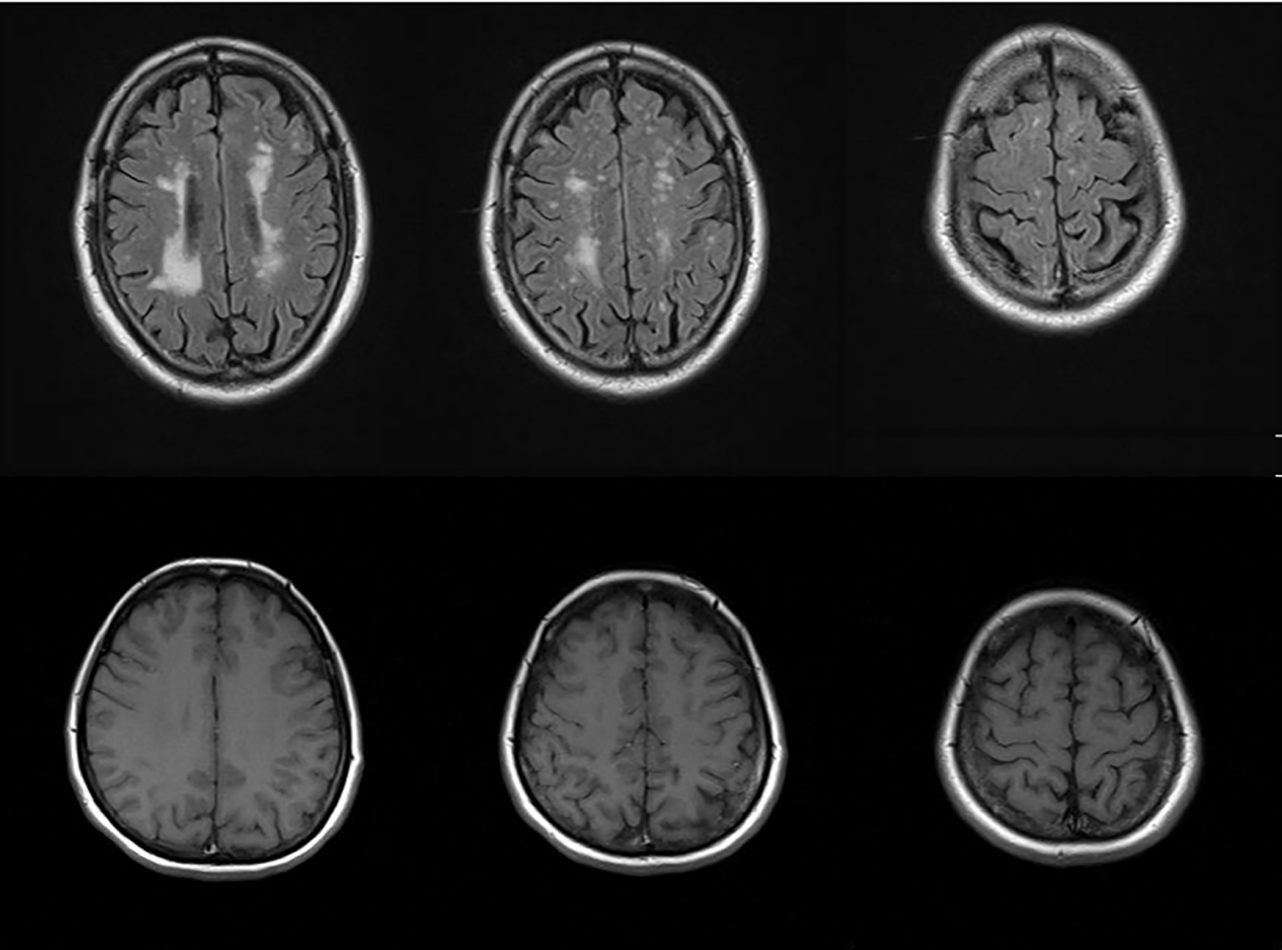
Typical examples of the presence or absence of cerebral white matter lesions. The upper three images are those of a subject with cerebral white matter lesions, while the lower three images are those of a subject without cerebral white matter lesions. T1 weighted images (T1WI), T2 weighted images (T2WI), and Fluid Attenuated Inversion Recovery (FLAIR) images were obtained using MRI equipment (MAGNETOM Symphony and MAGNETOM ESSENZA; Siemens Healthineers Global), but only the FLAIR images are shown here.
